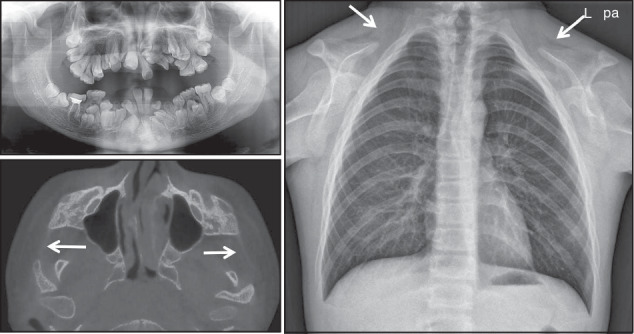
Cleidocranial dysplasia: the panoramic radiograph shows delayed exfoliation of deciduous teeth, retention of permanent teeth, supernumerary teeth, and the CBCT image shows discontinuous zygomatic arch, the chest radiograph shows hypoplastic clavicles
